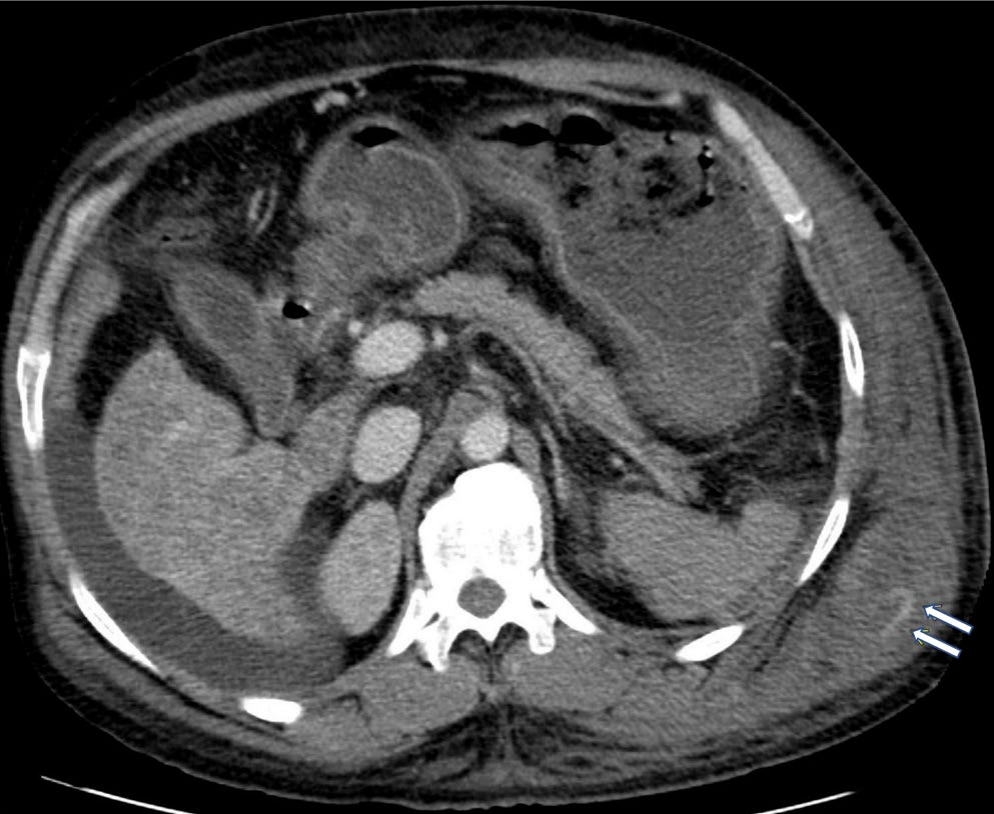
A computed tomography of the chest and abdomen in the axial view showing foci of high attenuation in the intramuscular hematoma involving the left lateral chest wall suggestive of acute bleeding, denoted by white arrows
